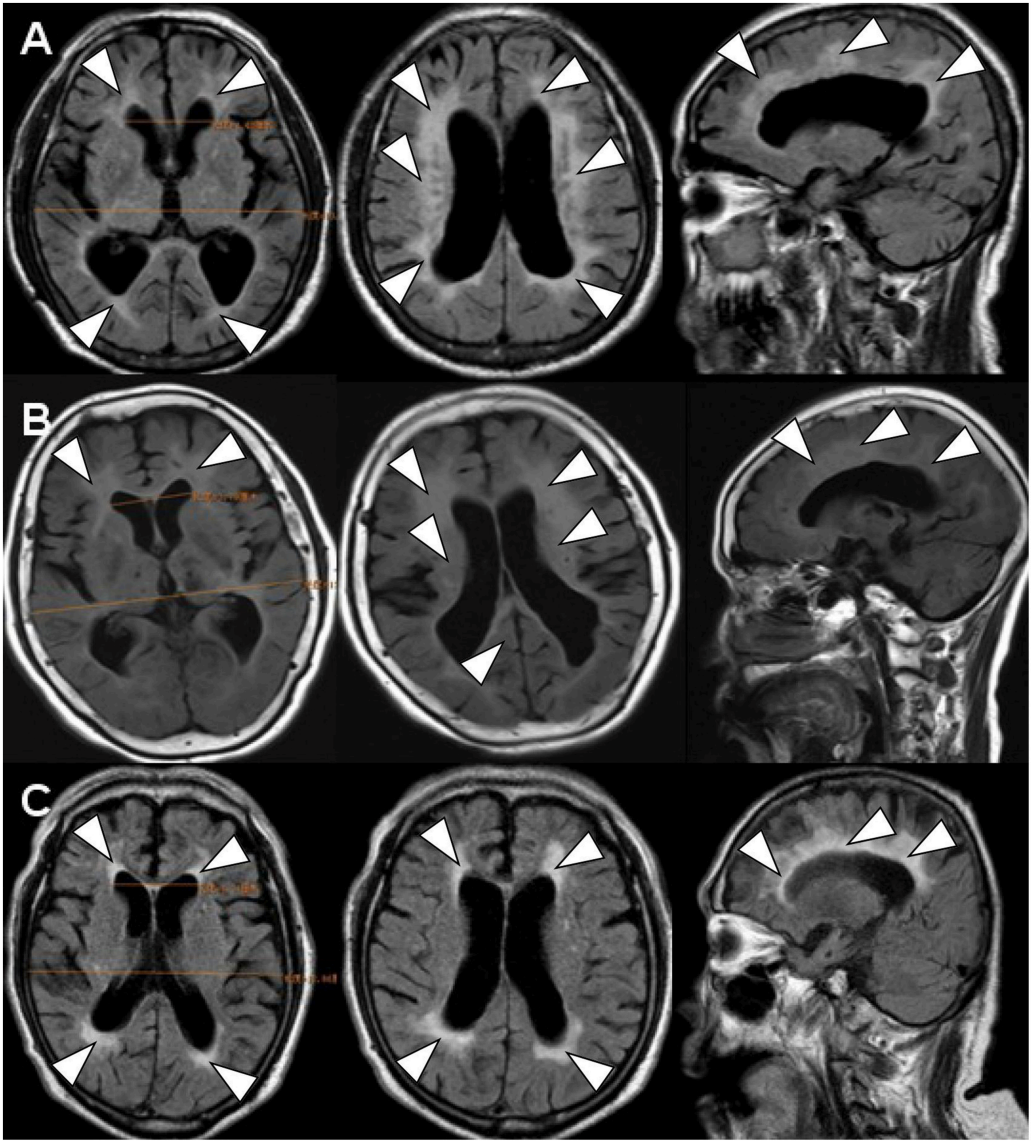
The brain FLAIR-MR imaging. FLAIR-MRI demonstrated the similar image feature of ventricular dilation (Evan index >0.3) (1) and periventricular (2) and deep white matter changes (3) in all of three cases. (A) denoted as INPH (case 1); (B) denoted as LA (case 2); (C) denoted as CAA (case 3).
